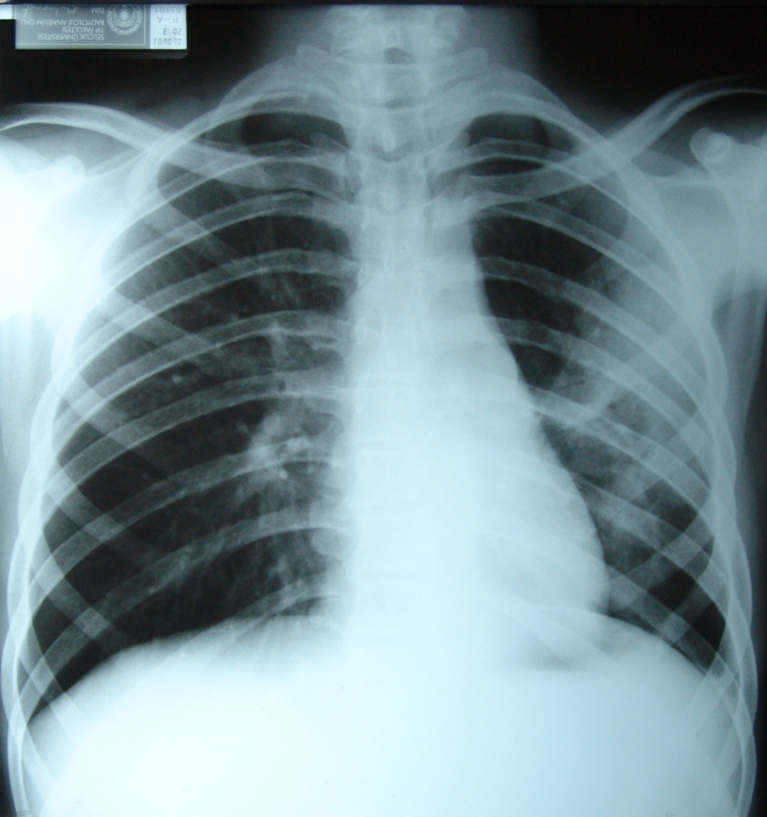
Lung radiography of the patient at admission showing pneumonia in the left lung and bronchial wall thickening on the right lung
